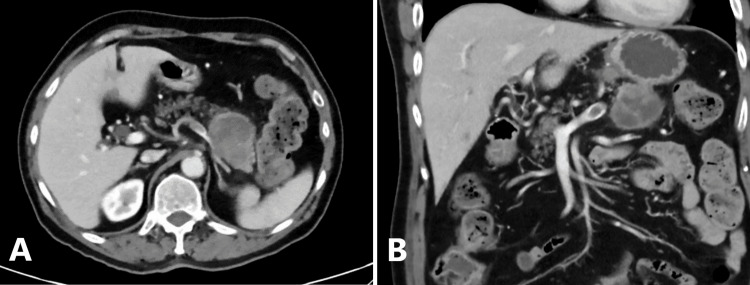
(A) axial and (B) coronal slices of CT at admission, demonstrating 50 x 59 mm (axial) mass of pancreatic body and tail. The tumor encased the splenic artery and was associated with pathological left gastric lymph nodes measuring up to 11 mm.
